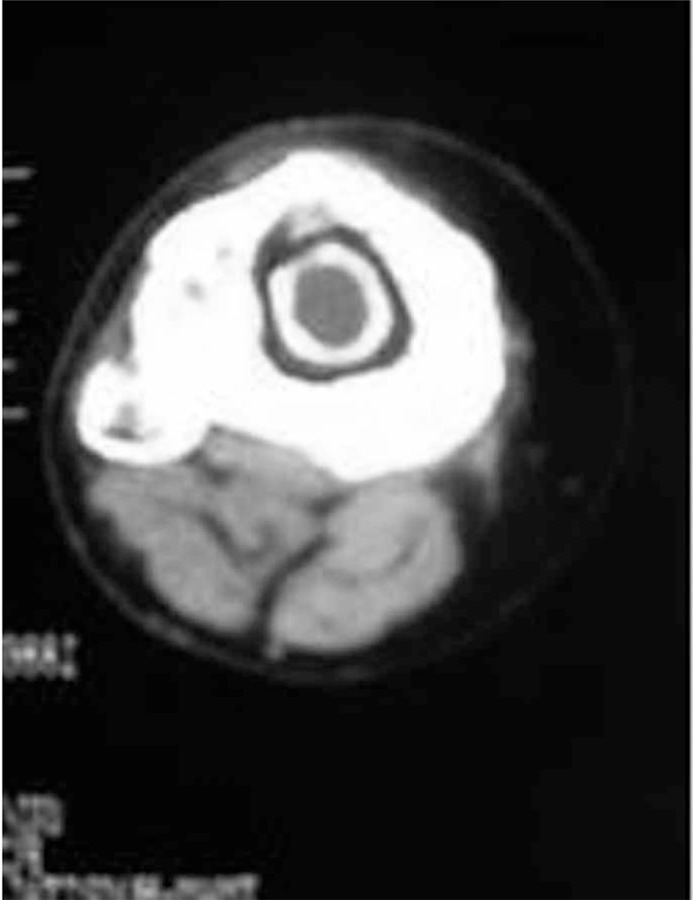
CT scan of the proximal left leg in the same patient shows a central intramedullary hypodense cystic lesion with a thick rim of ossification.
